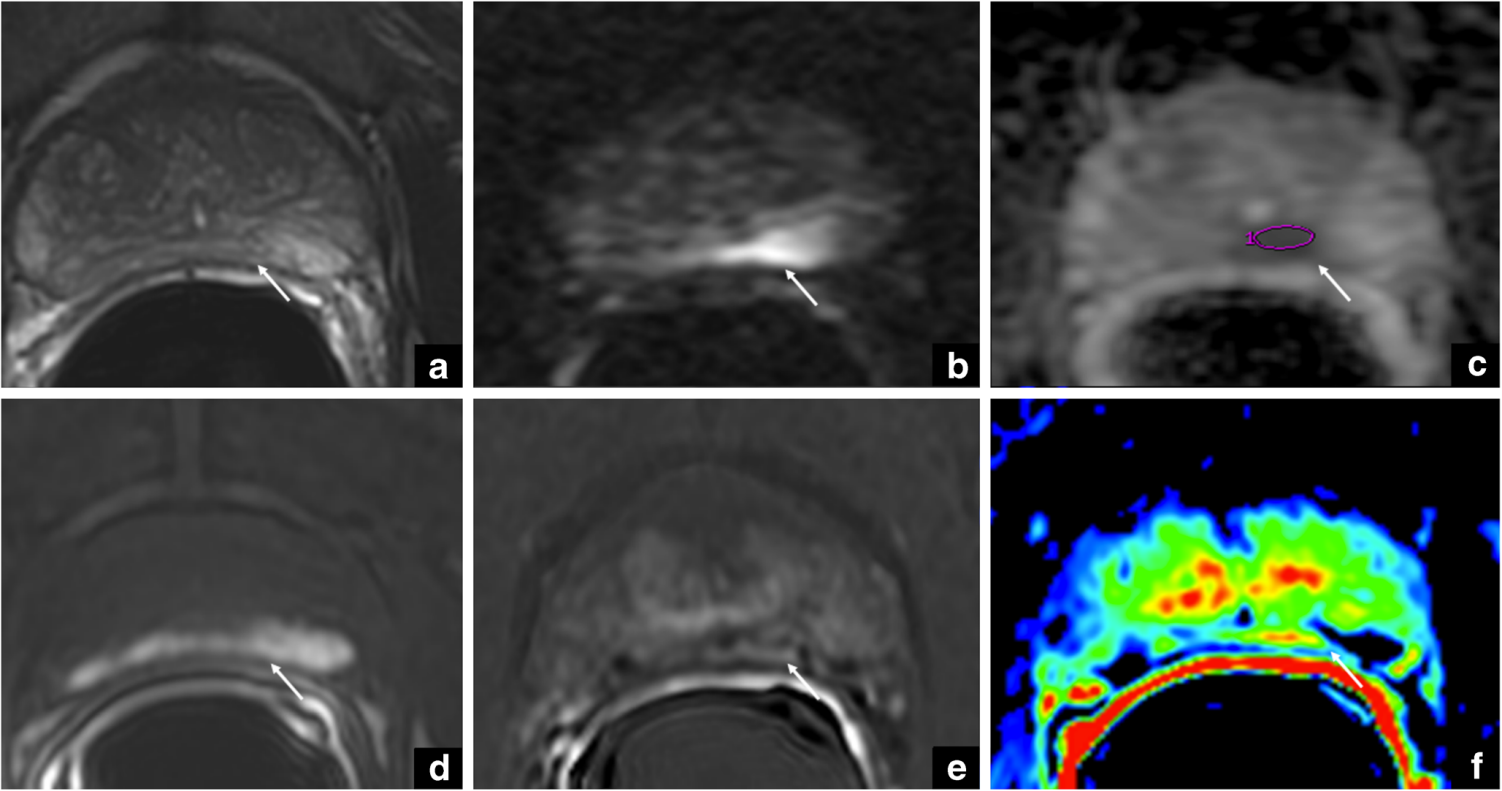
The arrows show an area of mild, low-signal intensity on T2-weighted imaging (a), with restricted diffusion on DWI (b) and in the ADC map (c). This corresponds to a hyperintense area on pre-contrast T1-weighted imaging (d), which is consistent with the products from the haemoglobin degradation after biopsy, as also supported by the post-contrast subtraction imaging (e) and in the colour DCE map (f). DCE studies have been obtained by gradient-echo sequences (TR: 4,5 ms; TE: 1,5 ms; flip angle: 15°; Average: 4; slice thickness: 2 mm; Matrix: 320 × 320; Scan Time: 3.13 min), using a body-weight adjusted intravenous bolus of gadobutrol (Gadovist, 1 mmol/mL; Bayer Schering Pharma, Berlin, Germany). DWI parameters were: TR ≥ 3000 ms; TE ≤ 90 ms; slice thickness ≤ 4 mm, no gap; field of view:160–220 mm; in plane dimension ≤ 2.5 mm phase and frequency; b values for DWI were 0–500- and values ranging between 1000 and 3000 s/mm^2^. For ADC maps, if only two b values can be acquired, it is preferred that the lowest b value should be set at 50–100 s/mm^2^ and the highest should be 800–1000 s/mm^2^
